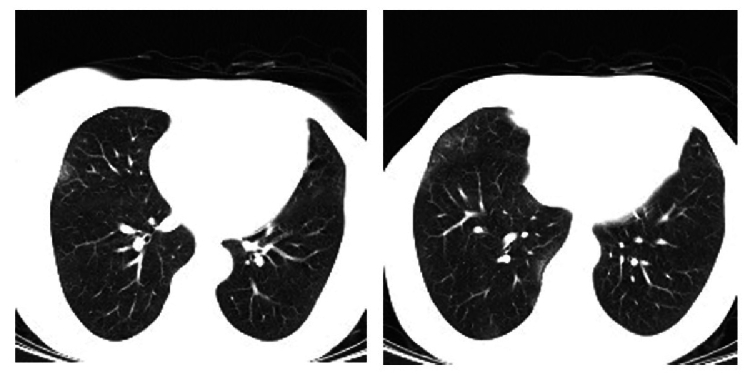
Axial chest CT shows small bilateral, multifocal peripheral and rounded ground glass opacities in the left image. The same pulmonary involvement is seen in another axial section, which is suggestive of SARS-CoV 2 infection.
